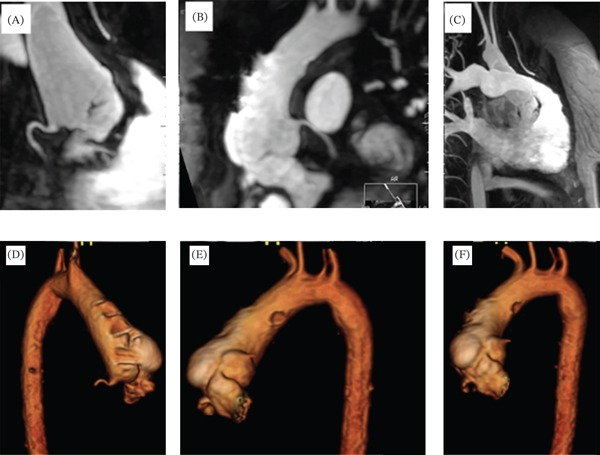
Cardiac MRI demonstrating anatomy of the aorta. (A) Origin of the right coronary artery. (B) Origin of the left coronary artery. (C) Relationship of the right ventricular outflow tract with the aorta. (D–F) Various three‐dimensional reconstructed views of the aorta/aortic root. Note the bulbous irregularities due to previous patch augmentation of the aorta.
